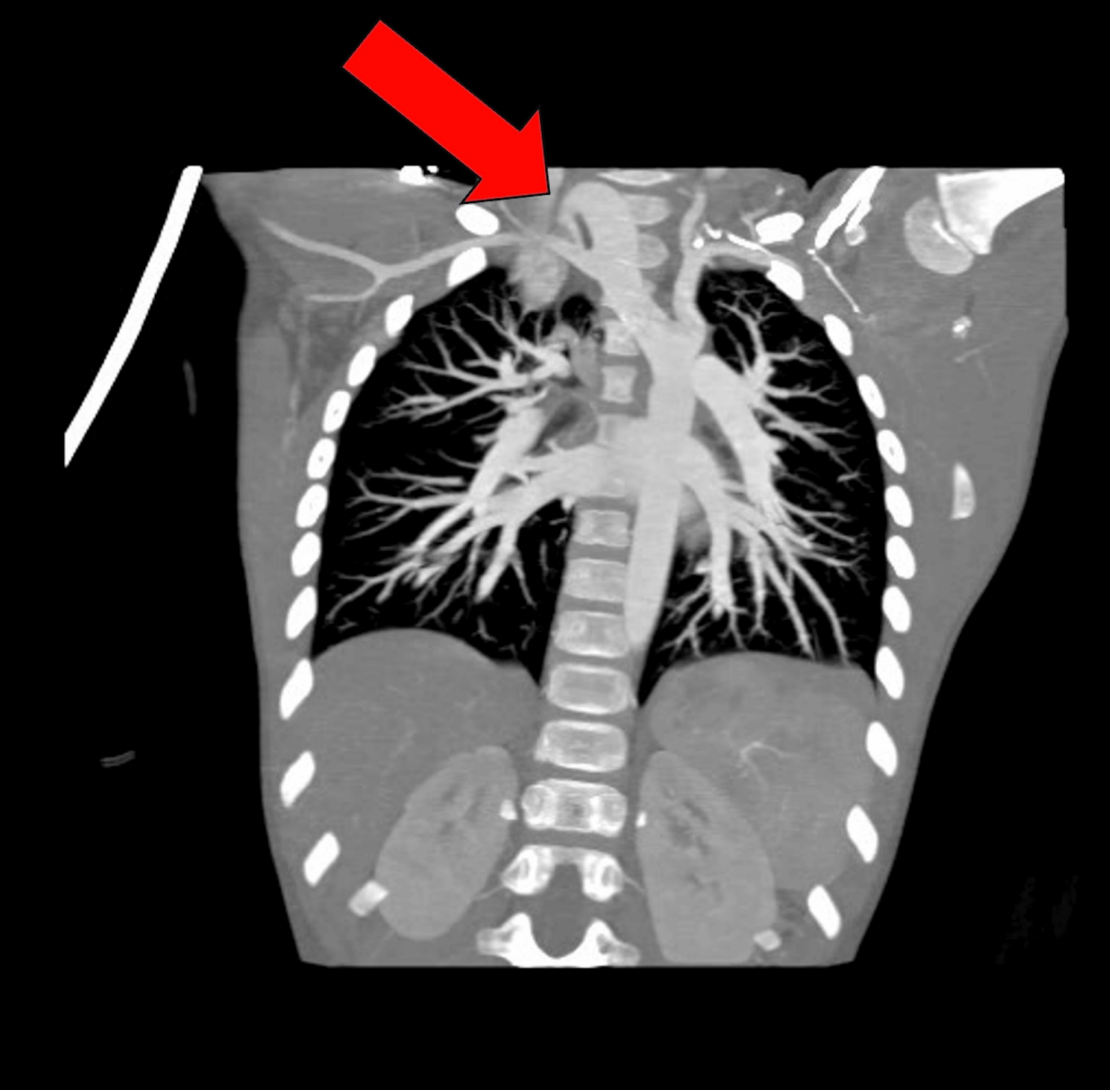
Coronal view from chest CTA The red arrow indicates the CAA on the right side of the body, contributing to VR formation. CAA, cervical aortic arch; CTA, CT angiography; VR, vascular ring
